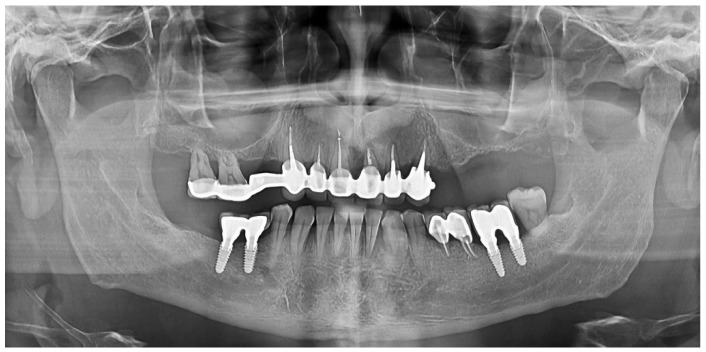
Patient’s radiograph at the beginning of SPT.
